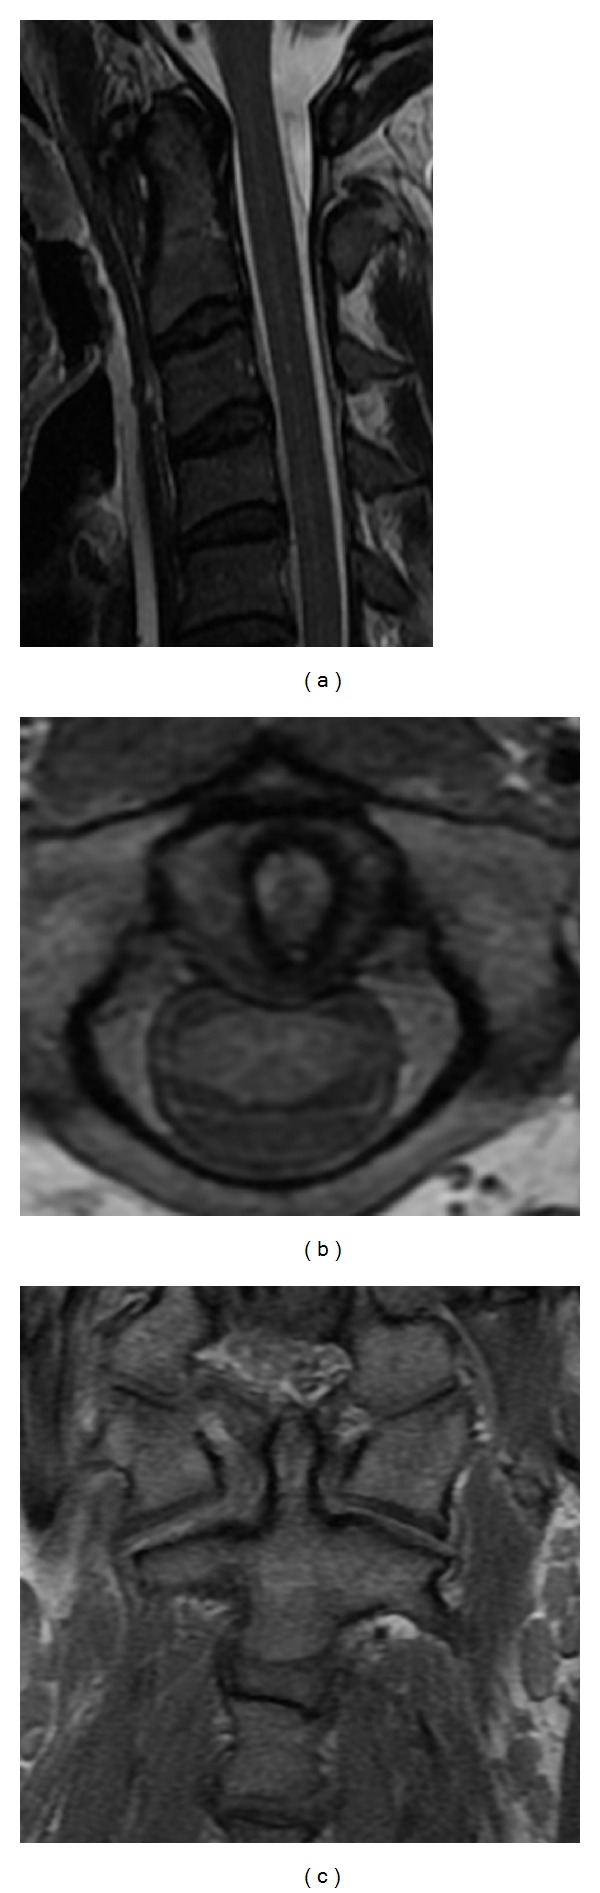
Cervical magnetic resonance imaging showing injury of ligament: midsagittal (a), axial (b), and coronal (c) view.
